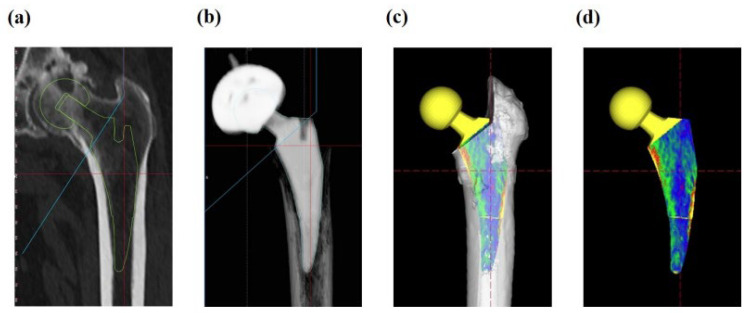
Fitting assessment of femoral component. Three-dimensional computed tomography (3D-CT) image (a) before and (b) 3 months after surgery. (c,d), Computer-aided diagnosis model of the placed stem reproduced in pre-surgery 3D-CT image based on the after-surgery information.
